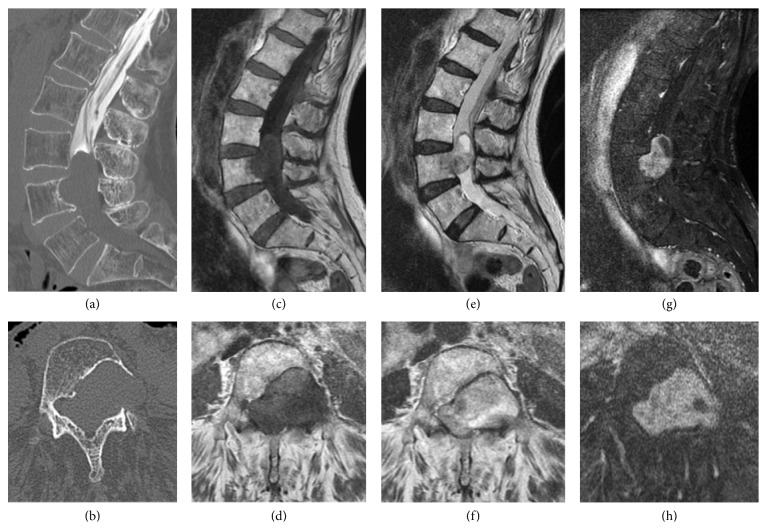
CT after myelography showing bone scalloping of the L4 vertebra body and left pedicle, and central spinal canal stenosis at L3 and L4 ((a) and (b)). MRI on the T1- ((c) and (d)) and T2-weighted images ((e) and (f)) before surgery. T1-weighted MRI scan after gadolinium administration showed enhancement of the spinal tumor ((g) and (h)).
